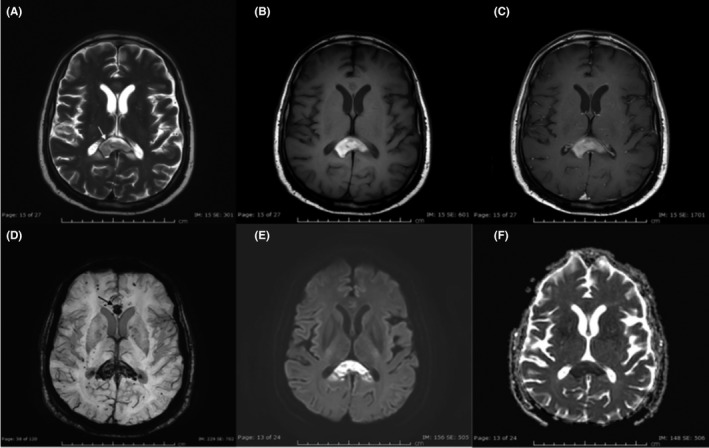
MRI brain axial T2W image of the brain (A) shows well‐defined hyperintense intraparenchymal hematoma in the splenium of the corpus callosum with mild perilesional vasogenic edema (white arrow). Pre‐ and post‐contrast axial T1W images of the brain (B and C) demonstrate hyperintense hematoma in the splenium of the corpus callosum with no post‐contrast enhancement. Axial susceptibility‐weighted image (SWI) of the brain (D) reveals blooming in the splenial hematoma and another hemorrhagic lesion in the genu of the corpus callosum (black arrow), and there are also multiple microhemorrhages in the cortical‐subcortical regions of bilateral cerebral hemispheres. Axial diffusion‐weighted image and corresponding ADC map of the brain (E and F) show diffusion restriction in the subacute splenial hematoma
